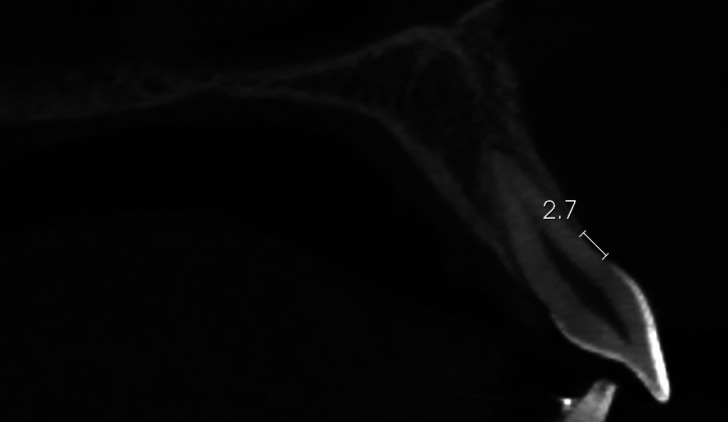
Measurement form CBCT: distance from the most apical portion of CEJ to the most coronal aspect of the buccal marginal bone crest, parallel to the long axis of the teeth.
